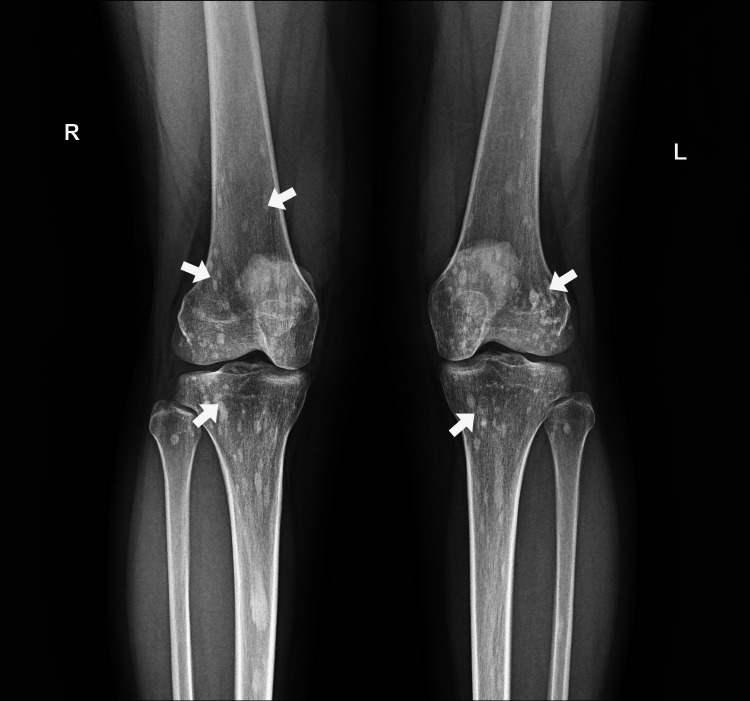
X-ray AP view of bilateral knee joint showing multiple lesions White arrows show multiple lesions in the bilateral knees in a symmetric pattern; the small, well-defined, ovoid/sclerotic nature of the lesions shows periarticular distribution. AP: anteroposterior; R: right lower limb; L: left lower limb
